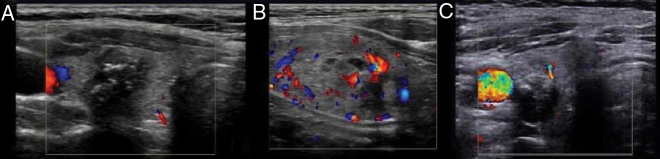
Subjective color Doppler ultrasound diagnosis. A, Avascularity; B, Vascularity ≤ 25% of the nodule circumference; C, Flow pattern is greater than that of the surrounding part.
